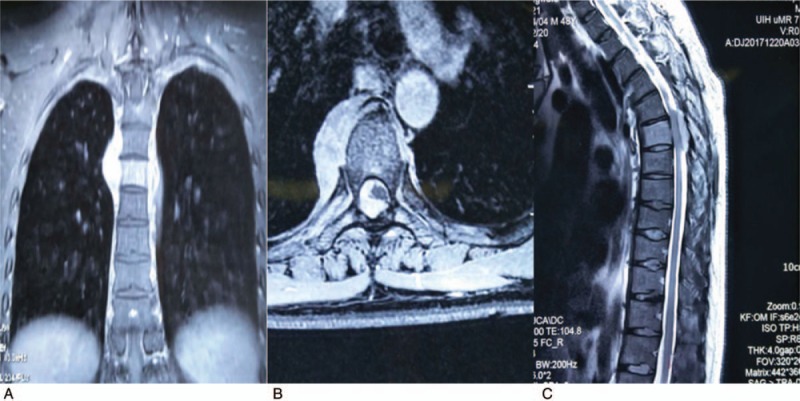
(A–C) MRI revealed an abnormal signal in the sixth thoracic vertebra with corresponding paravertebral and intraspinal occupying lesion that was considered as a suspected case of neurofibroma or hemangioma (A: coronal view, B: axial view, C: sagittal view).
